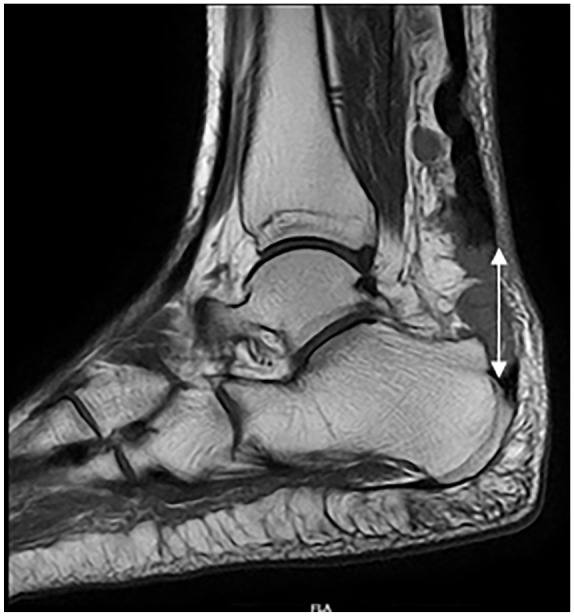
MRI right ankle shows full thickness tear of the tendoachilles with a gap of 3.4 cm.
